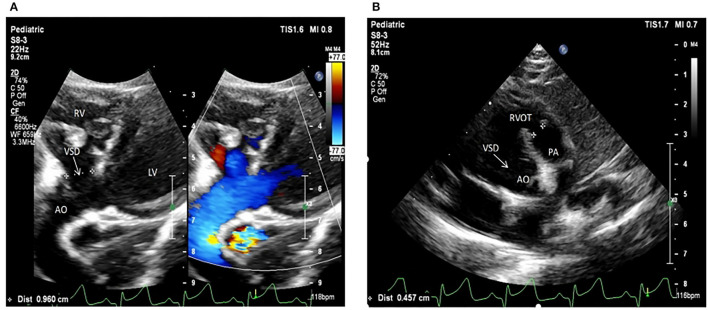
Echocardiogram images from patients II:2 and II:3 showing the apex of the heart. (A) The ultrasonogram for the elder twin (II:2). (B) The ultrasonogram for the younger twin (II:3). LV, left ventricle; RV, right ventricle; Ao, aorta; RVOT, right ventricular out ow tract; PA, pulmonary artery.
